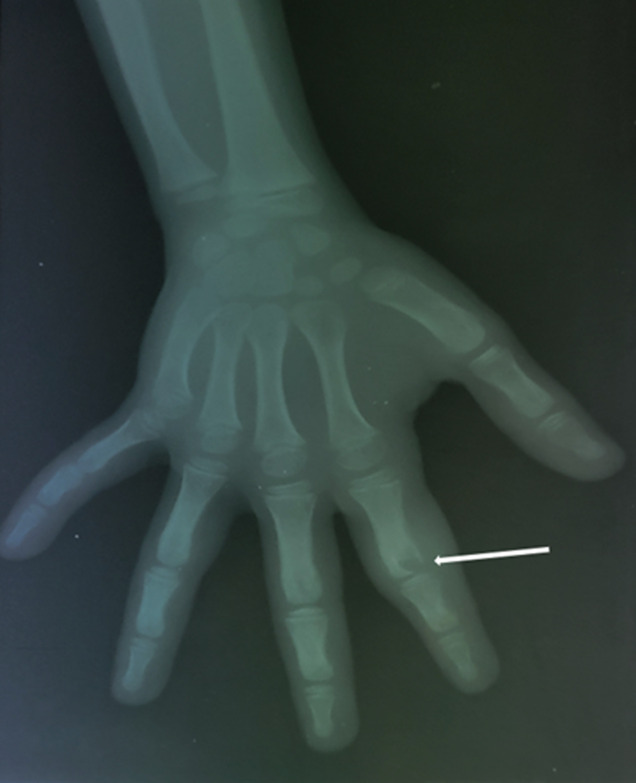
anteroposterior plain radiograph of the hand demonstrating an eccentric lytic lesion of the head and the neck of the proximal phalanx of the index finger (white arrow)
